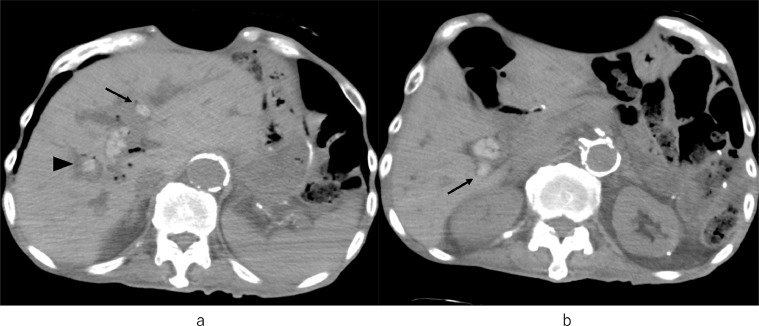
(A) Abdominal plain CT shows multiple stones in the left (arrow) and right anterior hepatic ducts (arrowhead) with biliary dilatation. (B) Abdominal plain CT shows multiple stones in the common hepatic duct and aberrant right posterior hepatic duct (arrow).
